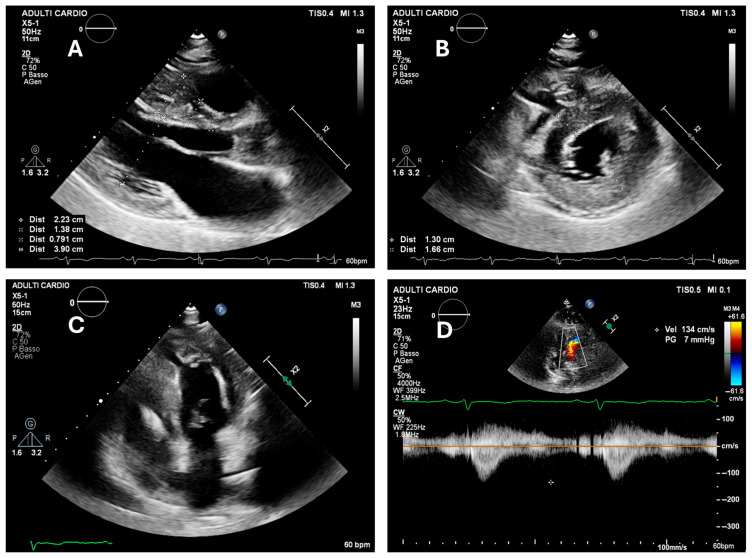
Transthoracic echocardiogram from a young pregnant woman with non-obstructive hypertrophic cardiomyopathy. (Panel A): Parasternal long-axis view showing marked septal hypertrophy. (Panel B): Parasternal short-axis view at mid-ventricular level, demonstrating severe hypertrophy of the inferolateral wall. (Panel C): Aneurysmal appearance of the true apex, with mildly reduced global systolic function due to diffuse hypokinesia. (Panel D): No significant intraventricular gradient detected at rest or following the Valsalva maneuver (* indicates a peak gradient of 7 mmHg).
